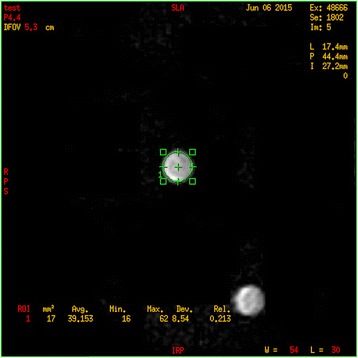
Axial T1-weighted imaging of colorectal cancer (CRC) cells after MnCl_2_ incubation. Regions of interest were manually placed to include the entire cell pellet area
